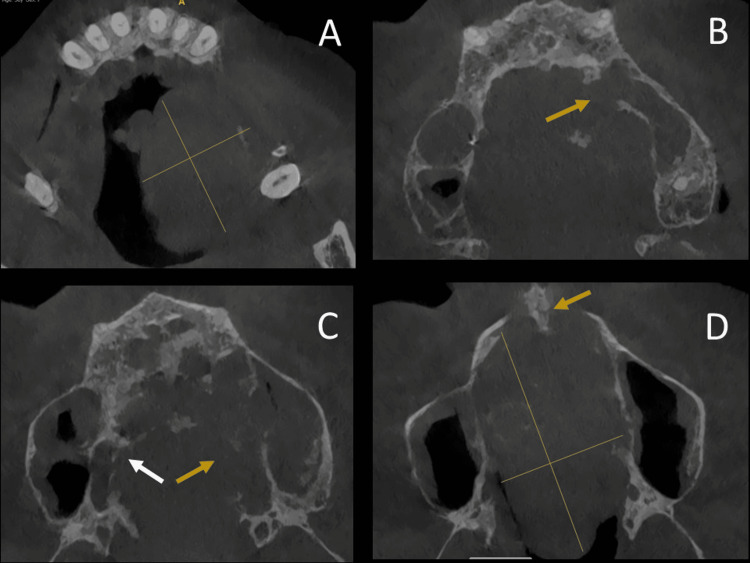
CT scan A: CT scan revealing the involvement of the superior left alveolar process. (The yellow cross shows the tumor’s extent.) B: CT scan revealing the involvement of the left and the right maxilla. (The yellow arrow shows the erosion of the left maxillary sinus medial wall.) C: CT scan revealing the involvement of the maxilla and both left and right maxillary sinuses. The lesion is crossing the midline. (The yellow and white arrows show the involvement of the left and right maxilla respectively.) D: CT scan revealing the involvement of the lower part of the nasal septum (as shown by the yellow arrow). (The yellow cross shows the tumor’s extent.) CT: computed tomography
